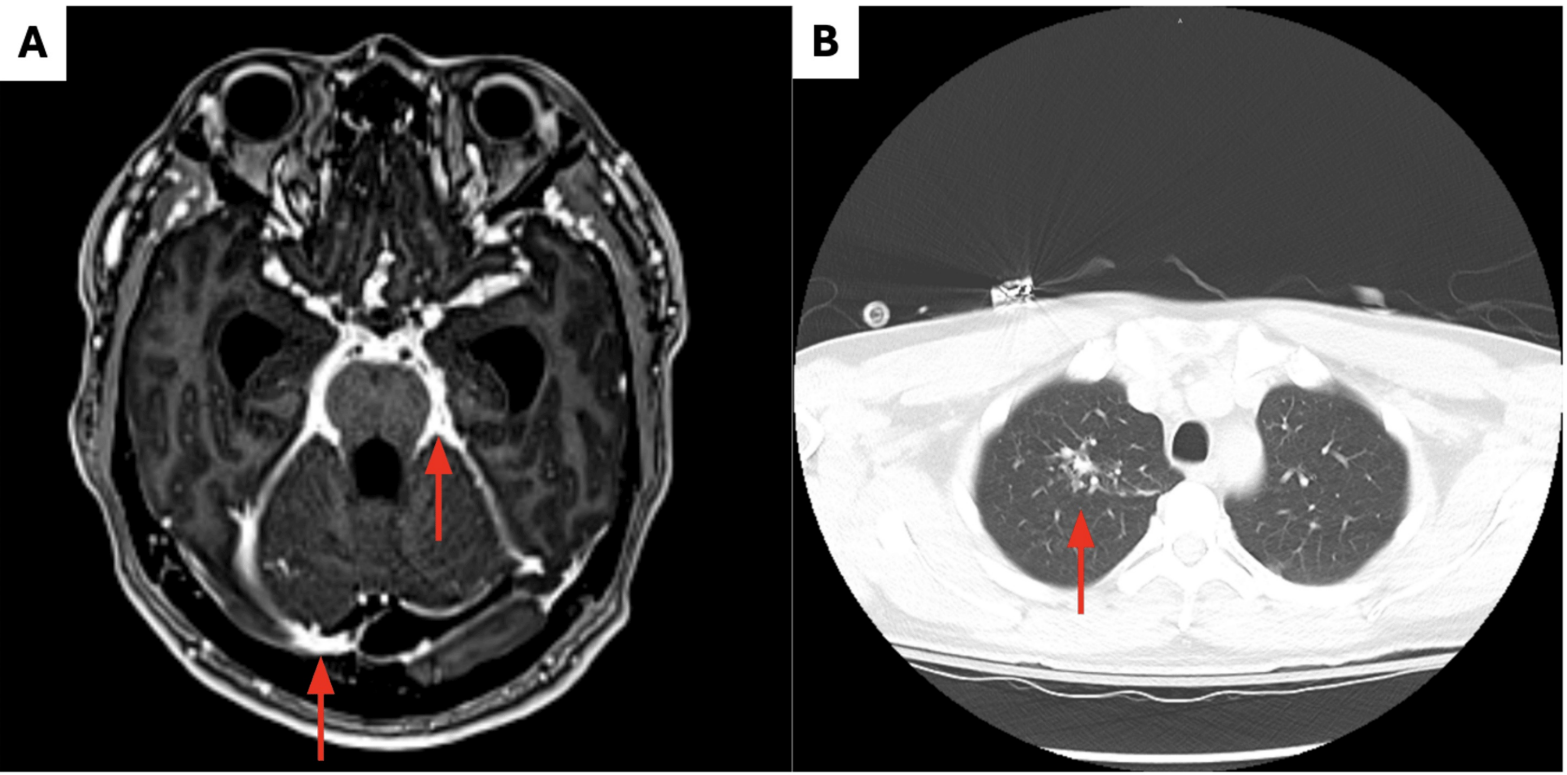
Patient imaging studies. (A) Axial T1 contrast MRI indicating leptomeningeal enhancement and subdural abscesses (arrows). B) Chest CT with right superior lobar nodular opacities in a tree-in-bud pattern (arrow).
